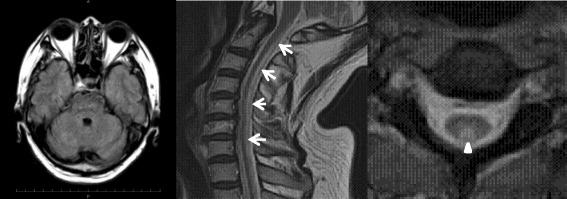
Magnetic resonance imaging of the patient. Brain axial MRI was normal, including images of the cerebellar white matter. However, cervical sagittal (*arrows*) and axial (*arrow head*) T2-weighted MRI revealed a high intensity area in dorsal columns of the C2-C7 spinal cord
